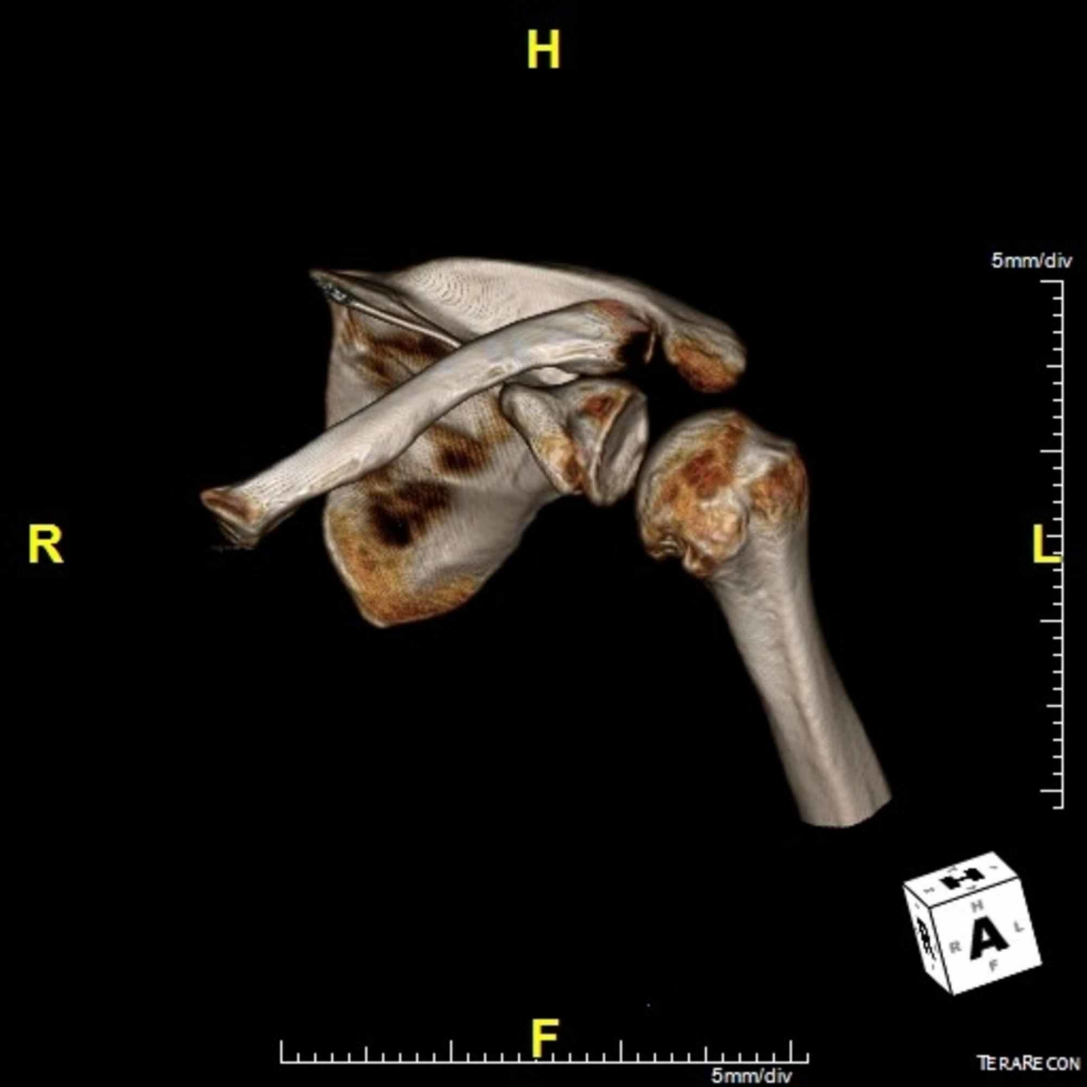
CT 3D reconstruction of the left shoulder again showing a large reverse Hill-Sachs deformity CT: computed tomography; 3D: three-dimensional
